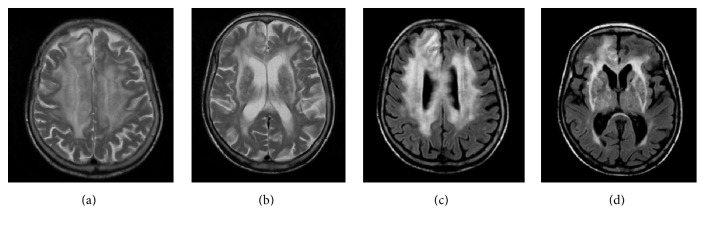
Axial T2 weighted (a, b) and FLAIR (c, d) MR imaging showing extensive white matter hyperintensity signal changes with a confluent pattern in periventricular and deep white matter of the cerebral hemispheres, without vascular distribution, more extensive on the right frontal region and involving all segments of the corpus callosum.
